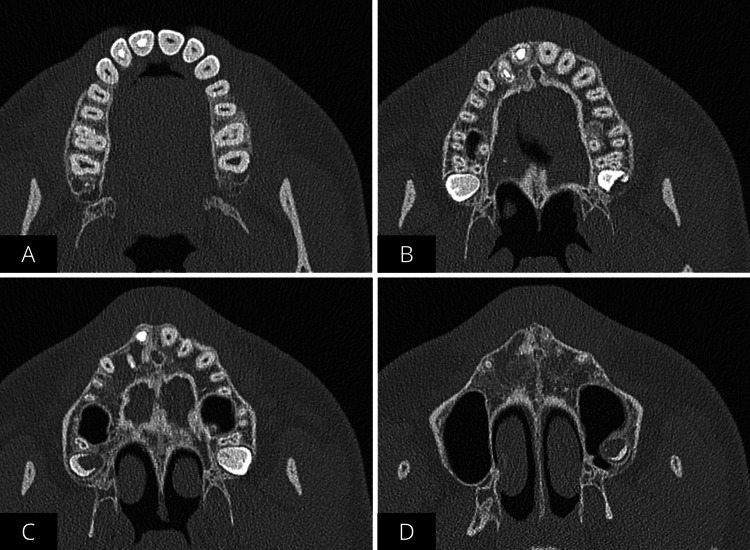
Final cone beam computed tomography showing progression of the axial view in which the complete healing of the cyst-like lesion and closure of the perforation of the buccal plate are appreciable Healing seen from the coronal aspect of elements 11 and 12 (A), middle third of elements 11 and 12 (B), apical third of elements 11 and 12 (C and D)
